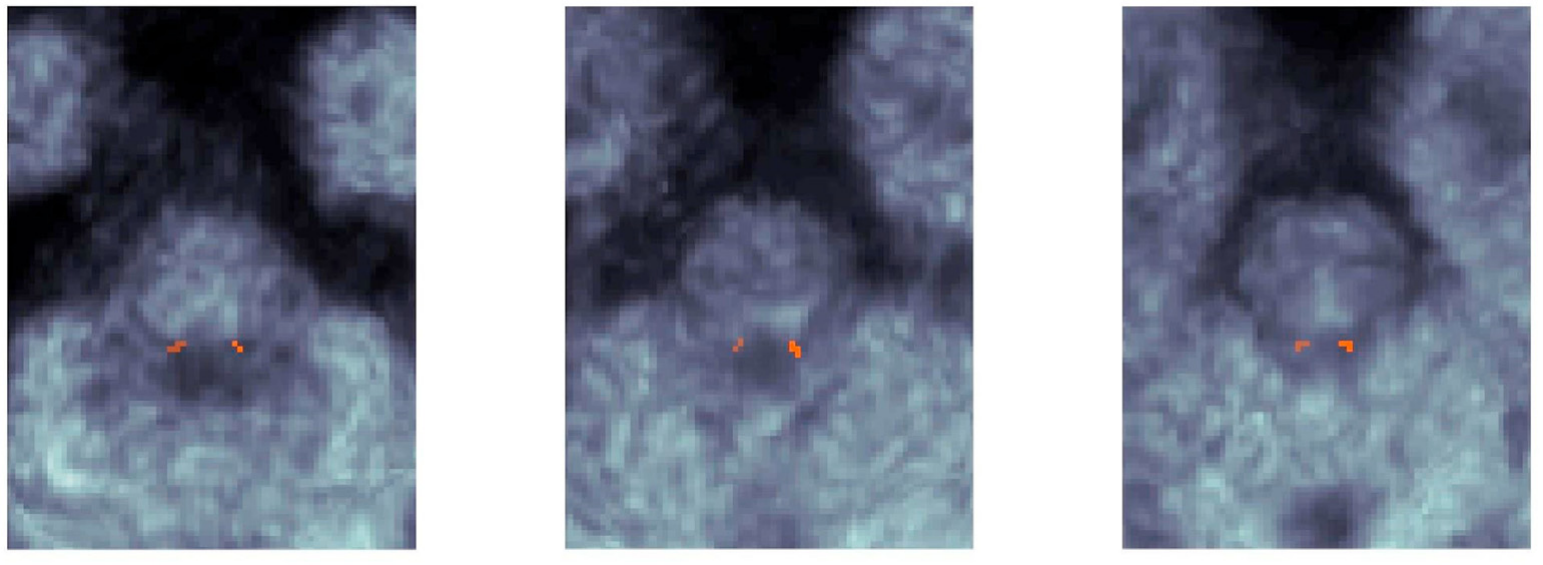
Example of the study co-registration accuracy protocol, which shows an individual’s co-registered FDG-PET images (at 3 different axial slices) and corresponding LC mask (Betts) position.
